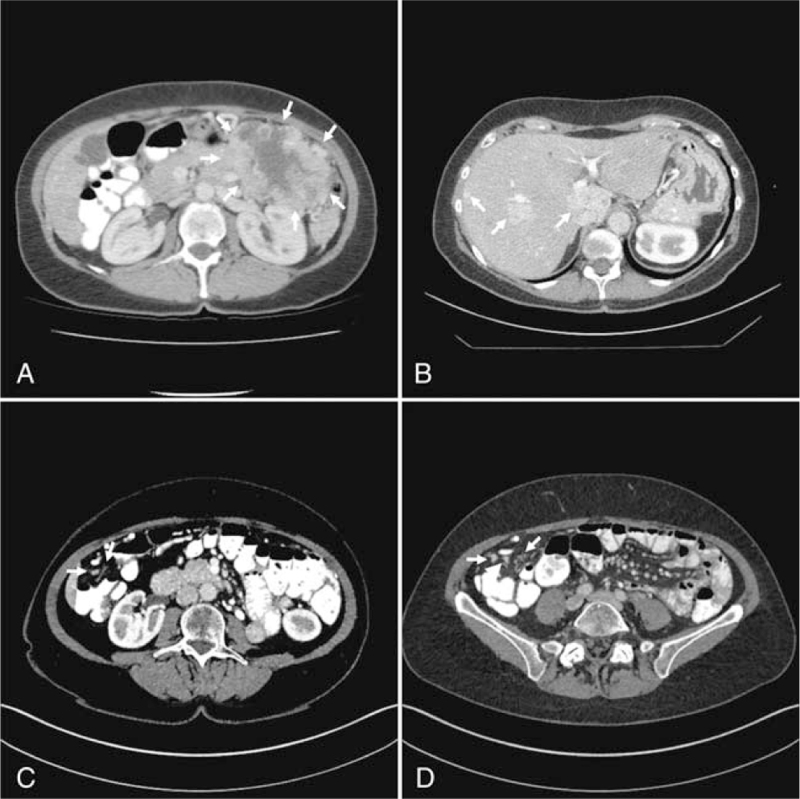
Initial and follow-up imaging of Case 3. (A) The initial contrast-enhanced CT of the abdomen revealed a large inhomogenous tumor bulk in the left upper abdomen affecting the left colonic flexure (white arrows). (B) The follow-up CT showed progressive disease with new liver metastases of the pNET in both liver lobes. (C) The follow-up CT of the abdomen demonstrated progressive peritoneal tumor manifestations in the upper right quadrant (white arrows). (D) The follow-up CT of the abdomen revealed progressive peritoneal tumor manifestations in the lower right quadrant. CT = computed tomography, pNET = pancreatic neuroendocrine tumor.
